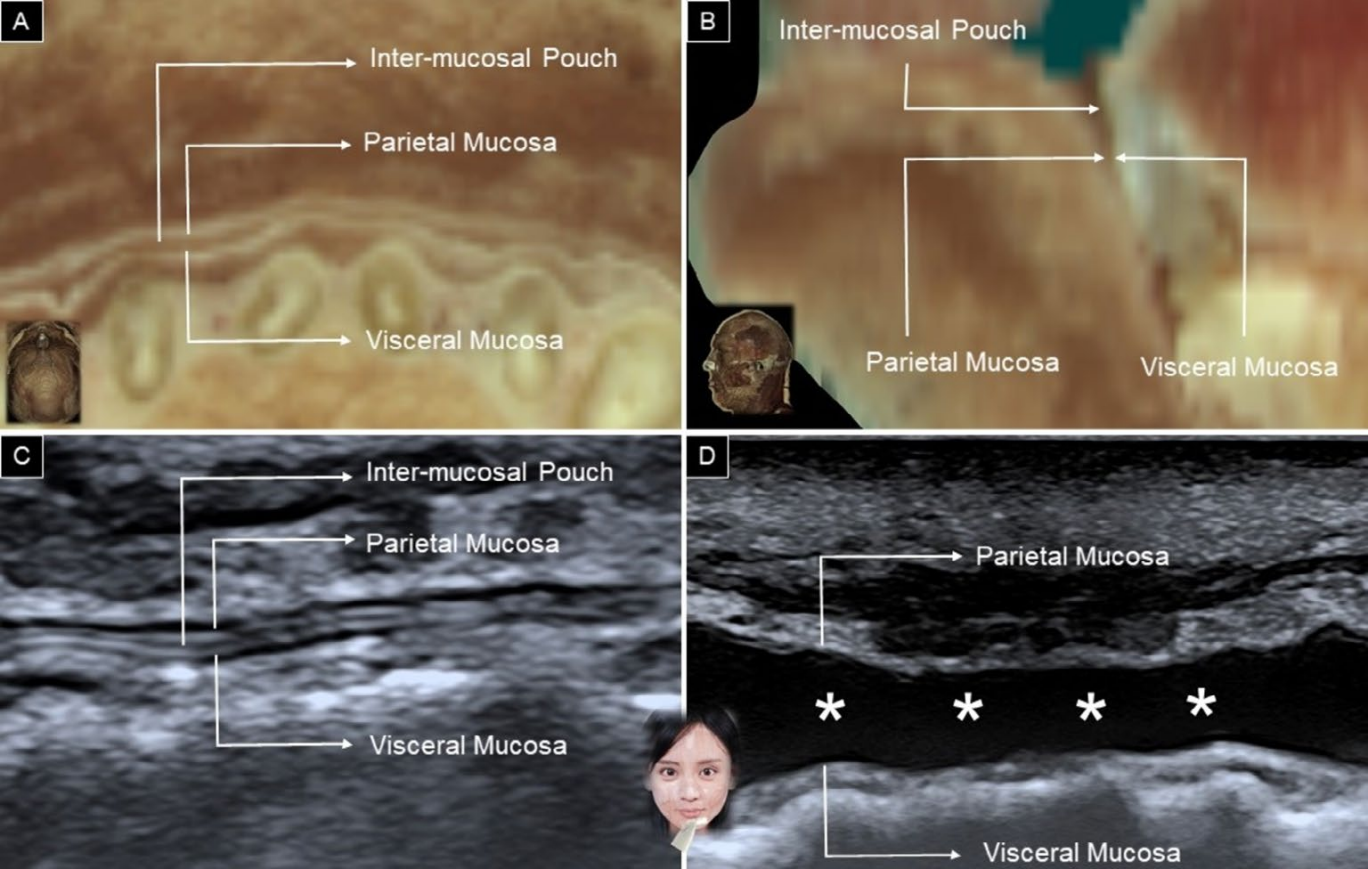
Cadaveric horizontal (A) and sagittal (B) dissections of the inter‐mucosal pouch, along with its ultrasound images in the horizontal plane before (C) and after (D) dilatation with water (asterisk). Cadaveric images are adapted from the Visible Human Project of the National Library of Medicine. Excerpts from these images, featured in the VH Dissector, are used with permission from Touch of Life Technologies Inc.
